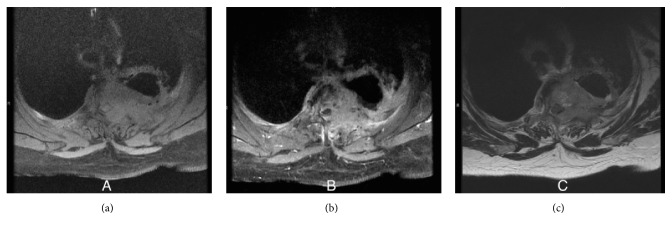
Magnetic resonance imaging (MRI) of the thoracic spine in the axial plane with (a) T1-weighted sequence without contrast, (b) T1-weighted sequence with contrast, and (c) T2-weighted sequence.
